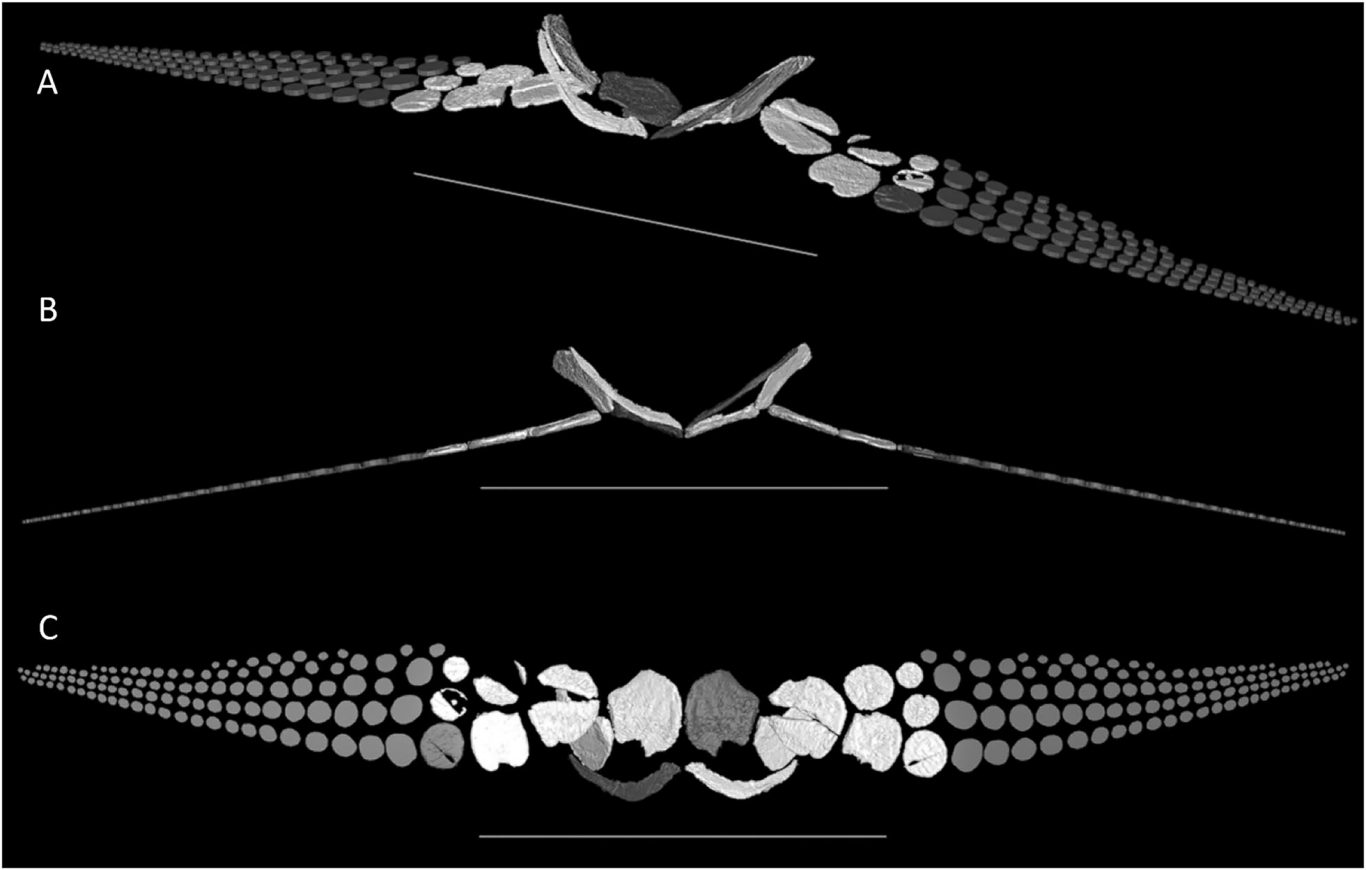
3D reconstruction of the scapular girdle and forefins of *Besanosaurus leptorhynchus* based on the CT scans of BES SC 999 in laterofrontal (A), frontal (B), and ventral (C) view. Original elements in white; elements reconstructed on the basis the available material in grey. Scale bars represent 50 cm. Note that the forefin elements were subject to extreme dorsoventral compression post-mortem and appear much more flattened in the reconstruction than they would be in vivo
